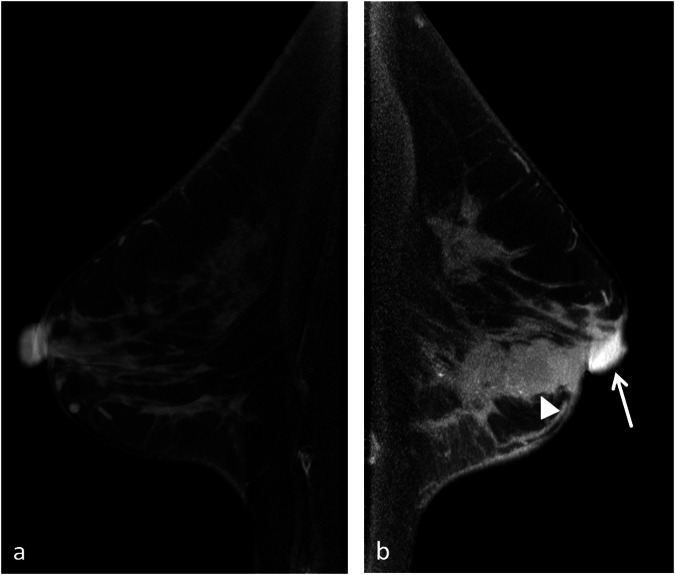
CE-CBBCT of a 58-year-old patient with invasive ductal carcinoma (arrowhead) in the left breast (b). In this patient, there was a conspicuous enhancement on the left nipple (arrow), while there was no enhancement on the right nipple (a), which was a typical example of asymmetric NAC enhancement. In addition, nipple retraction and periareolar skin thickening were observed. NAC involvement on the left nipple was confirmed by postoperative pathology. CE-CBBCT, contrast-enhanced cone-beam breast CT; NAC, nipple–areolar complex
